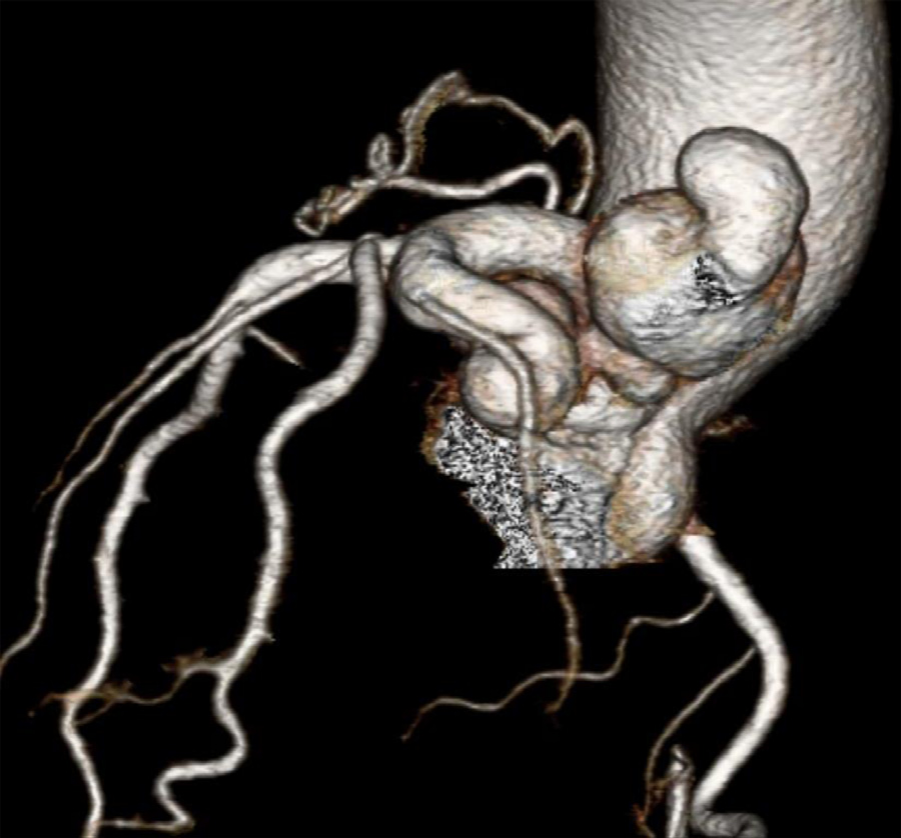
3D reconstruction from cardiac CTA: dilated and aneurysmal LCX CAF.
